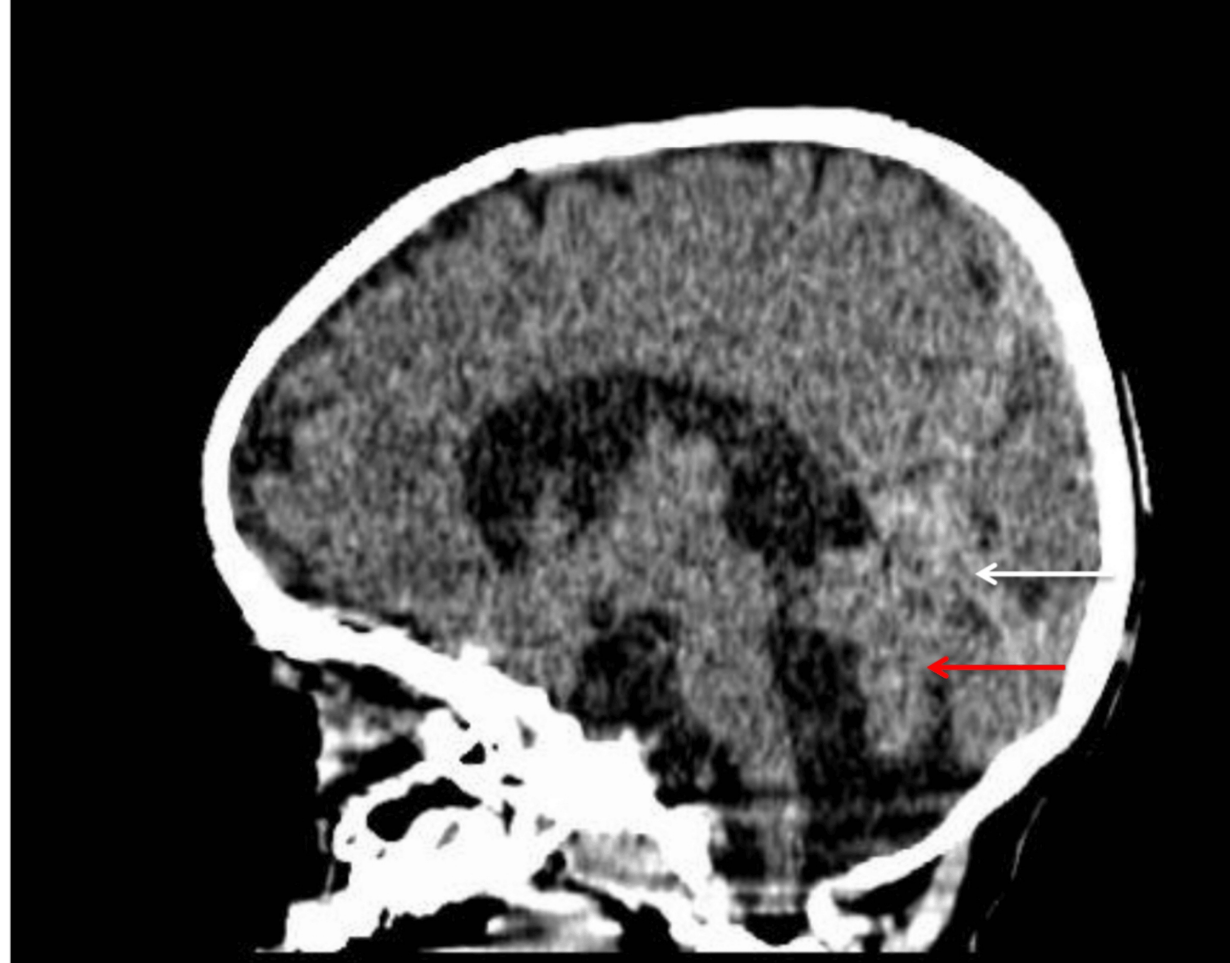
Sagittal section of the CT scan showing vermian hypoplasia (red arrow) associated with ascension of the cerebellar tent (white arrow)
